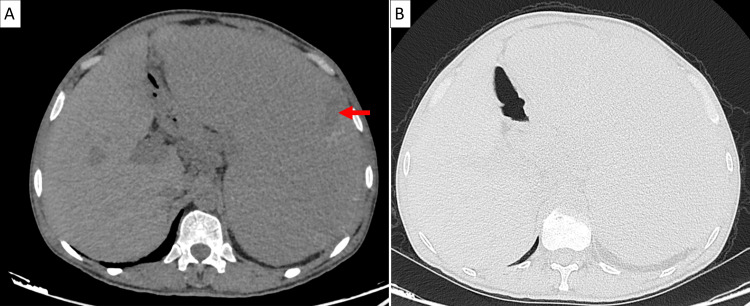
Abdominal sections from the CTCAP showing massive splenomegaly with a splenic infarction focus (arrow) CTCAP: chest-abdomen-pelvis computed tomographic scan (A) Transverse noncontrast section. (B) Transverse contrast-enhanced section
